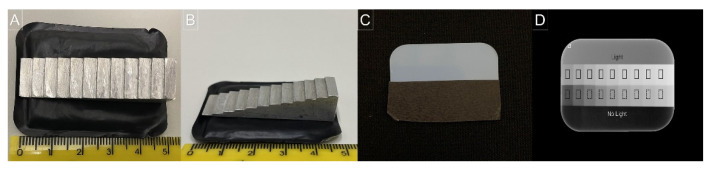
Setup for X-ray and light exposures. (A,B). Upper and lateral view of the aluminum step-wedge longitudinally fixed in the center of a sheathed size-2 PSP plate for X-ray exposure. (C). Upper view of the PSP plate with half of the sensitive surface covered with black paperboard for ambient light exposure. (D). Representative radiograph with the 18 ROIs: 9 in the exposed-to-light half (full-line rectangles) and 9 in the non-exposed-to-light half (dotted-line rectangles).
